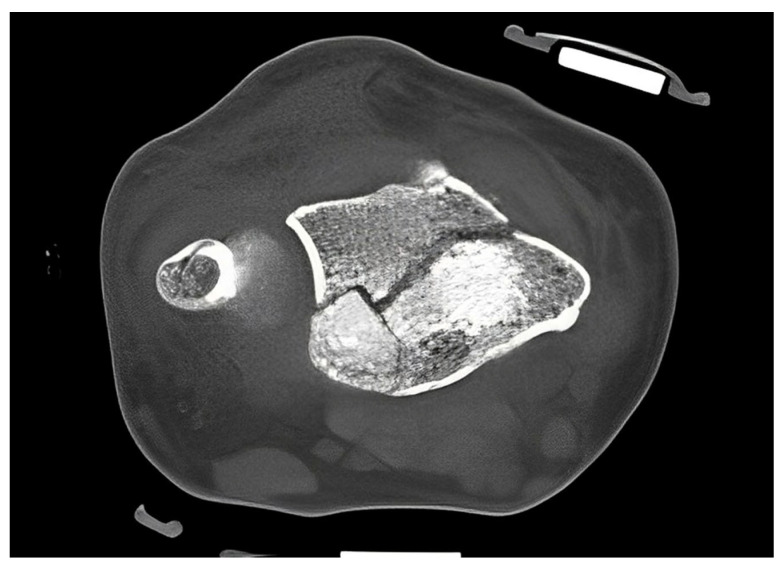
CT scan of a DRF in transversal view. The use of aluminum-based orthosis does not induce metal artifacts.
